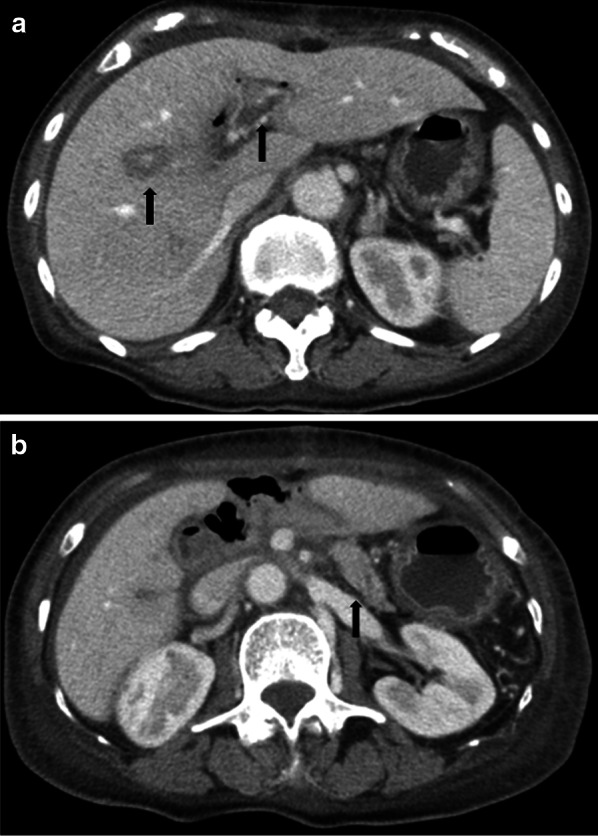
Postoperative contrast-enhanced computed tomography (CECT) evaluation. a CECT on postoperative day 14 shows intrahepatic portal thrombosis (black arrow) and edema of the Glissonian pedicle, while the superior mesenteric vein anastomosis was patent without thrombosis. b No evidence of intraperitoneal infection, such as pancreatic fistula or bile fistula, was observed. The black arrow indicates the remnant pancreas
